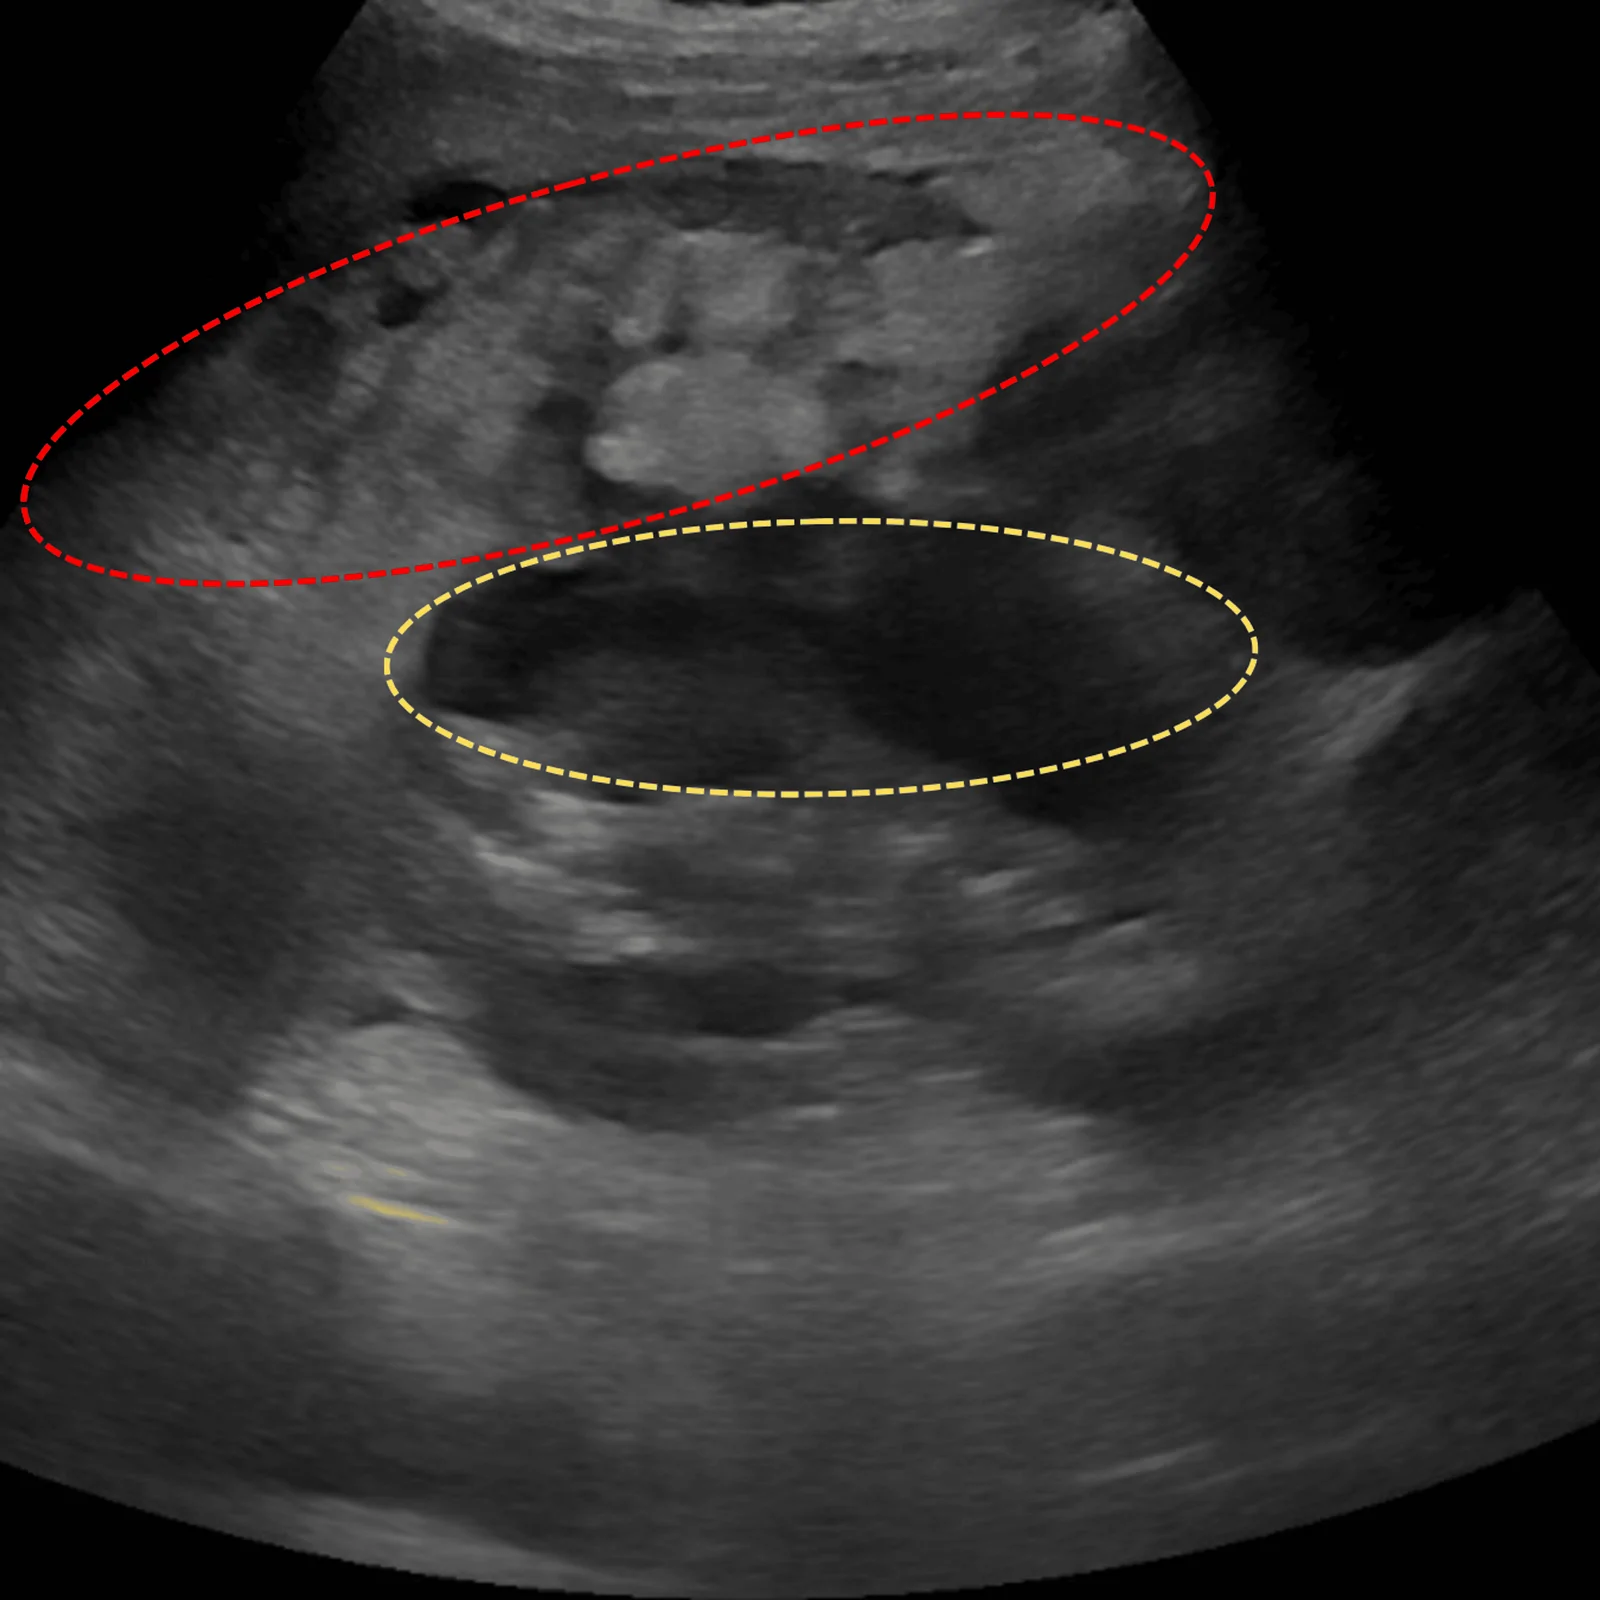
Abdominal ultrasound An echogenic heterogeneous mass with a polypoid and infiltrative appearance was identified occupying the right anterior pararenal space (red lines). Adjacent to the lesion, a subcapsular renal hematoma with an estimated volume of approximately 80 cc was observed (yellow lines). A contrast-enhanced abdominal computed tomography (CT) scan was recommended for further characterization.
